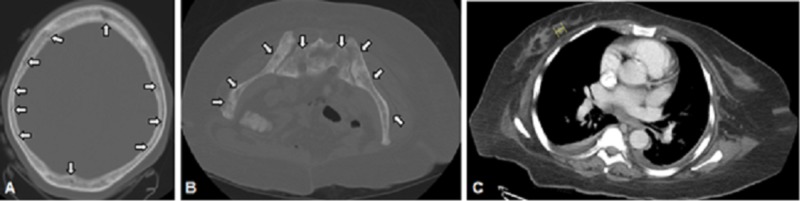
A) White arrows point to mixed lytic and sclerotic lesions of the calvarium found on computed tomography (CT) scan of the head without contrast. B) White arrows point to osteoblastic lesions of the pelvis found on CT scan of the abdominopelvic region without contrast. C) Yellow marker shows 1.2 cm ovoid soft tissue nodular opacity at the 6 o’clock position of the right breast.
